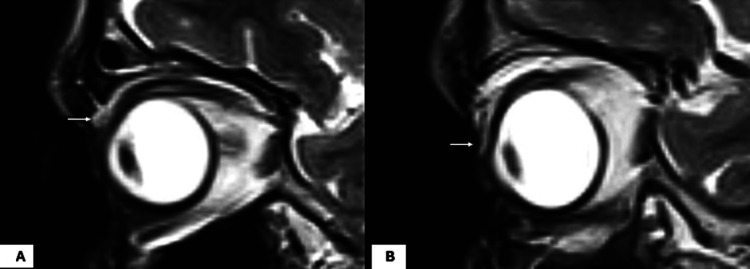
T2-weighted oblique-sagittal section of MRI orbit showing disinserted and retracted LPS aponeurosis in the right eye (white arrow in A) and the aponeurosis inserted into the upper border of the tarsal plate (white arrow in B) in the left eye.
